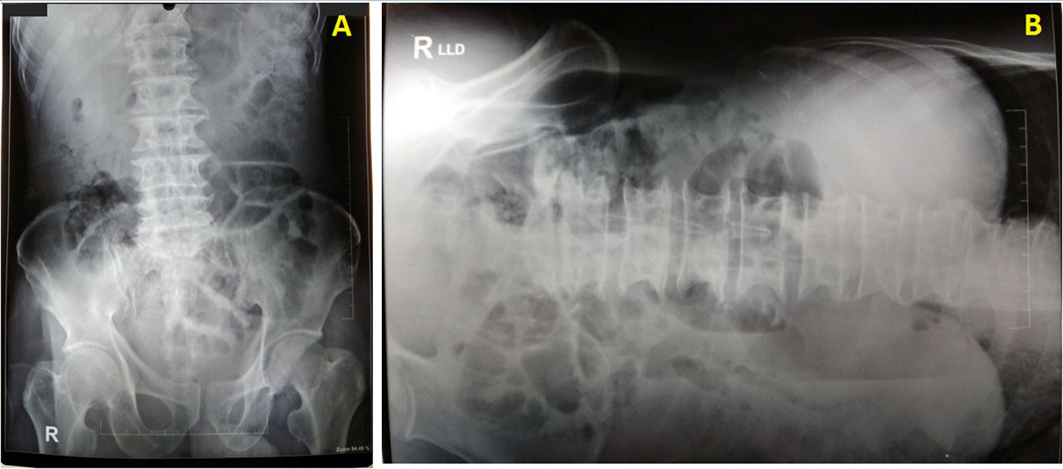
Abdominal X-ray evaluation (A) at supine position showed positive Rigler sign. (B) At left lateral decubitus position showed free air in peritoneal space.
